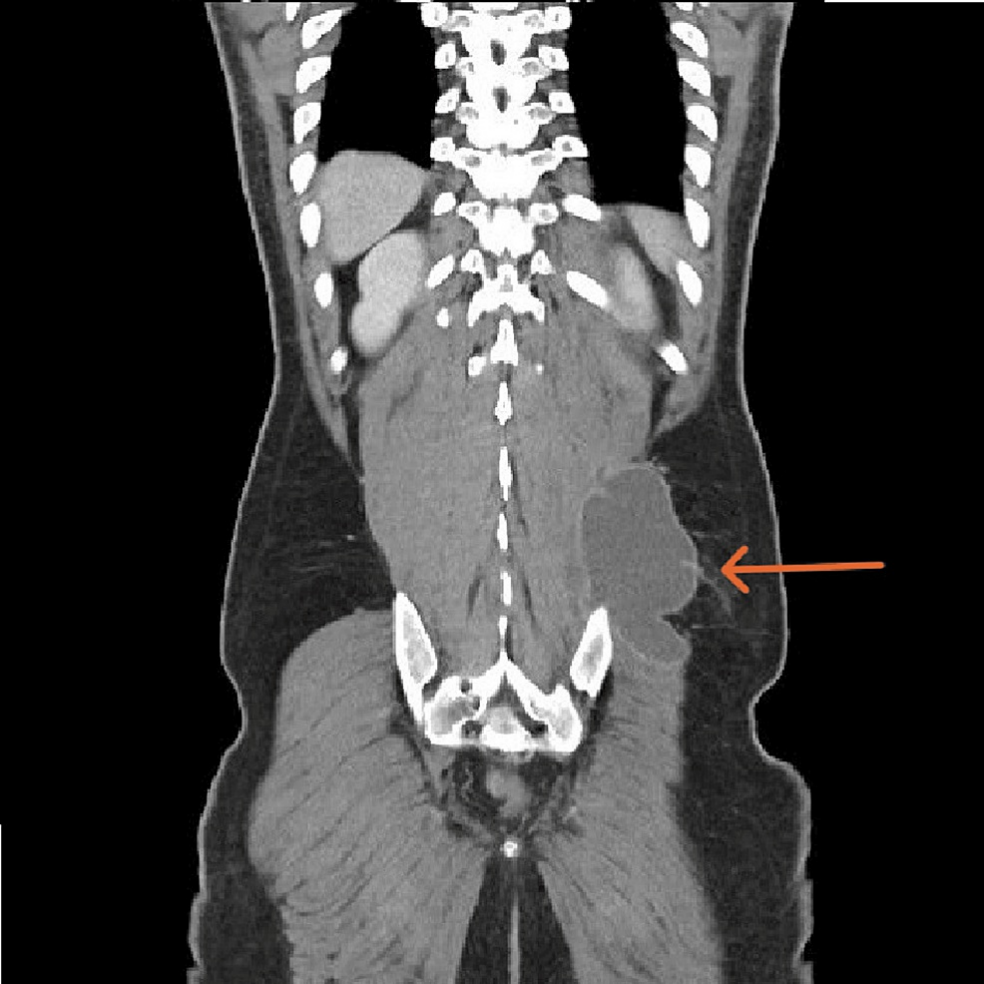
Coronal cut CT of the abdomen showing left gluteal/paraspinal collection measures approximately 12 cm × 6.5 cm, with anterior and posterior vertebral extension.
